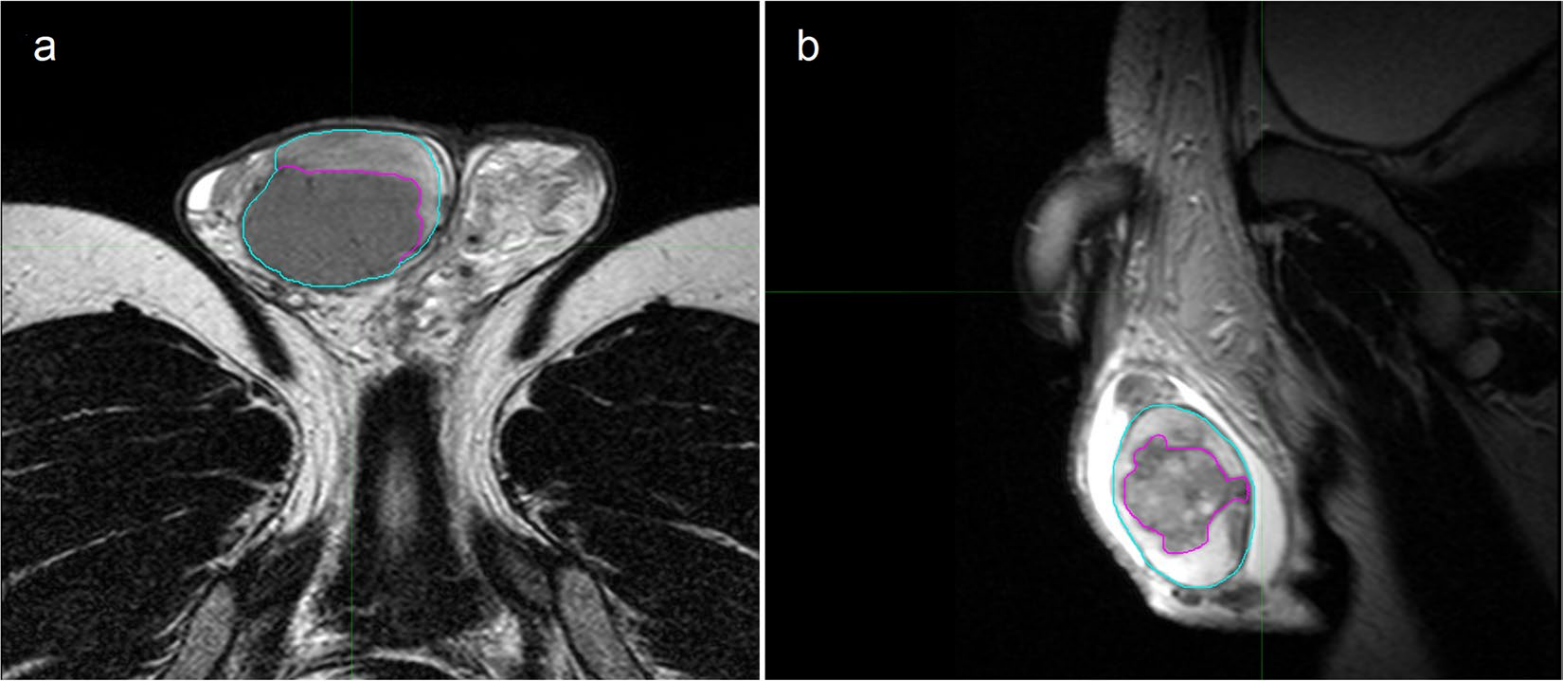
Examples of testicular lesions. (a) Testicular seminoma and (b) Germinal tumor. Axial and sagittal T2-weighted images, respectively. Testicles are contoured in cyan, whereas neoplasms are contoured in violet.
